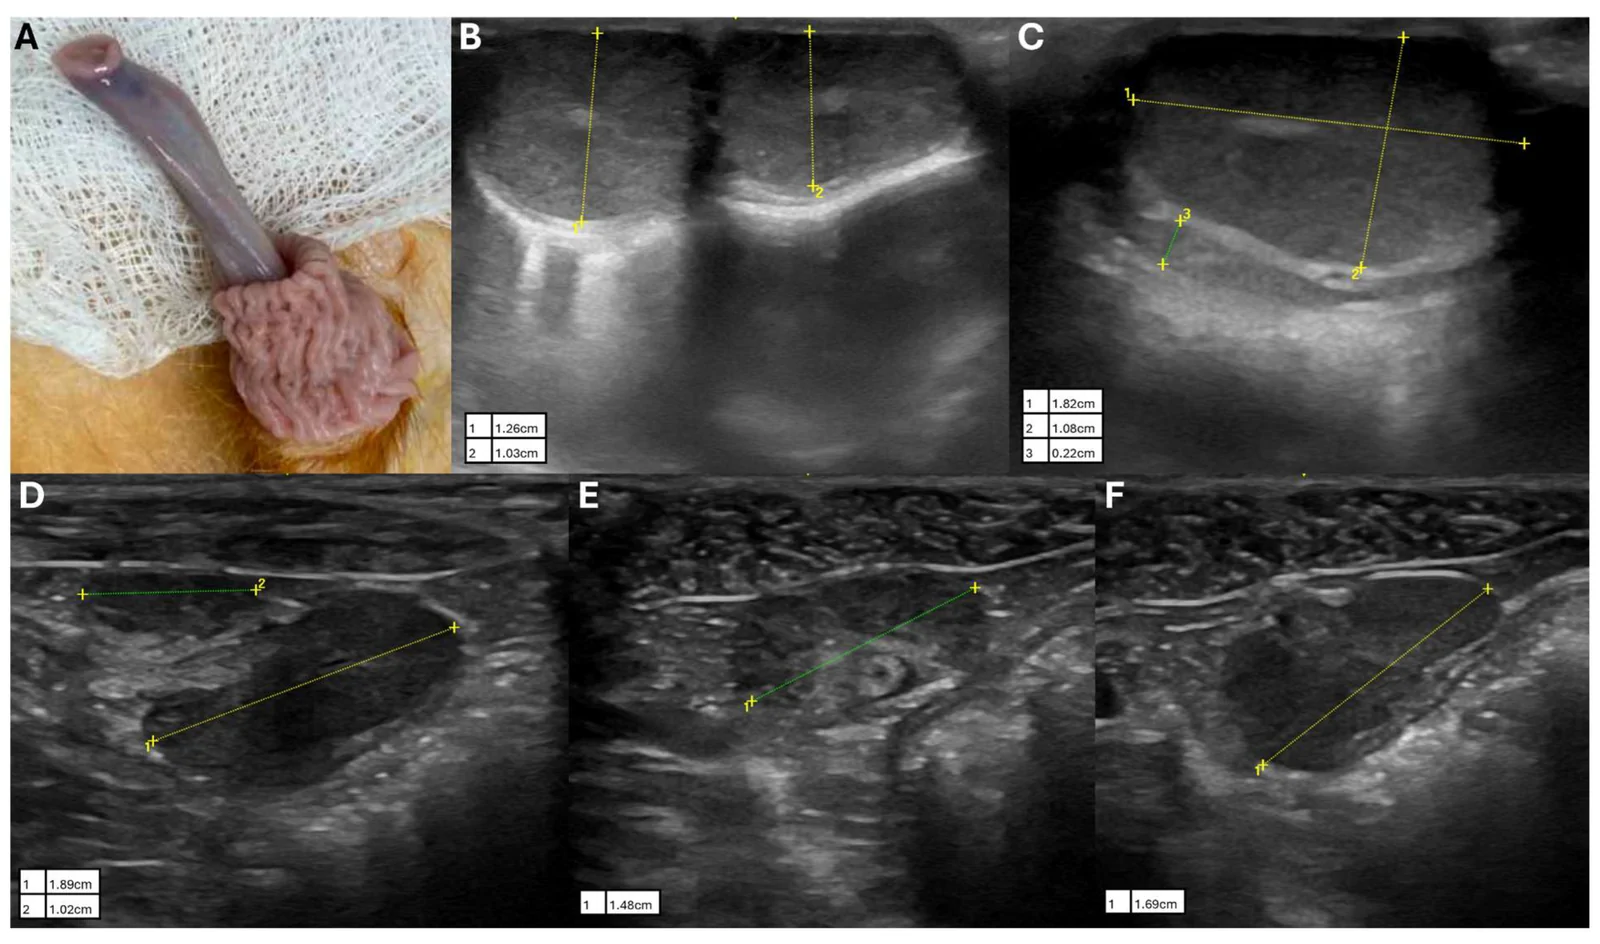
Macroscopic image of the (A) penis protruding out of the prepuce and sonograms of the (B) left and right testis (transversal view), (C) one testis and the epididymis (longitudinal view), (D) cranial and caudal prostatic lobes (longitudinal view), (E) cranial prostatic lobe (transversal view), and (F) caudal prostatic lobe (transversal view) of male 2. Yellow and green lines indicate length/width of the reproductive organ in cm. Numbers in tables correspond to the numbers within each image.
